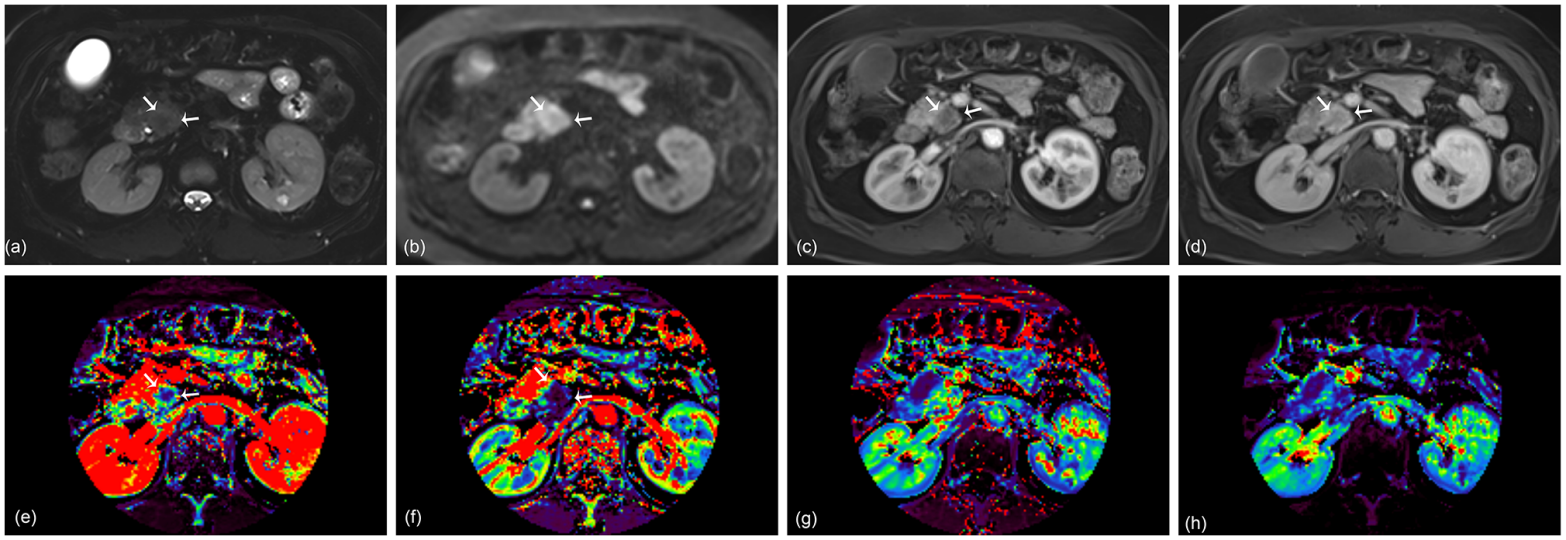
A 67-year-old woman with pancreatic ductal adenocarcinoma in the pancreas head. A 2.4 cm tumor shows high signal intensity on axial T2-weighted image (A) and diffusion-weighted image (B). Arterial (C) and portal (D) phase images of dynamic contrast-enhanced (DCE) MRI show no rim enhancement of the tumor. K^trans^
